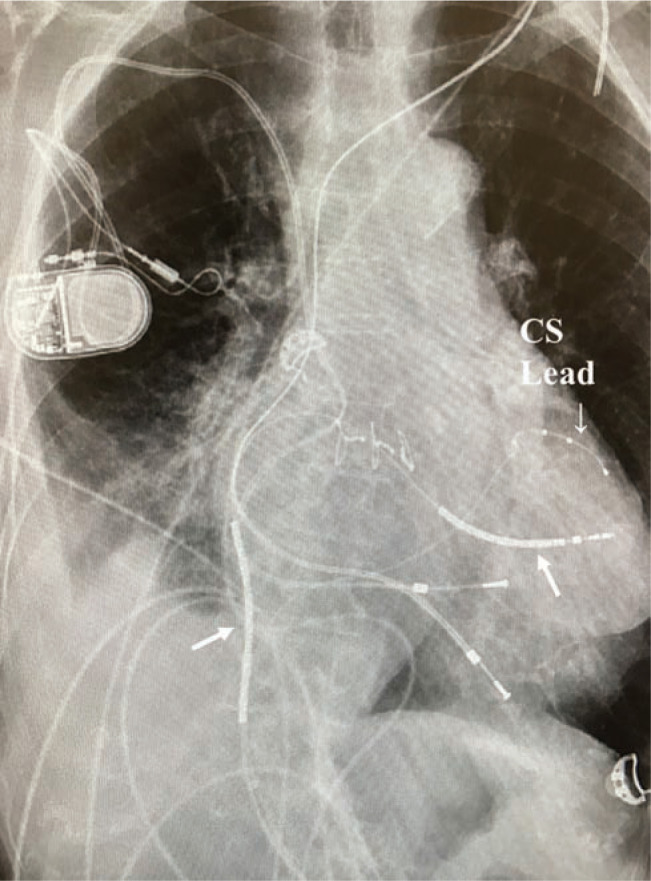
Postoperative posterioranterior chest radiograph showing two new transvenous leads inserted via the iliac vein. The CS lead is labeled, present in a basal posterolateral location. Note the insertion of the RV shocking lead in the mid-RV septum, chosen to separate the two defibrillation coils with RV myocardium between them (arrows).
